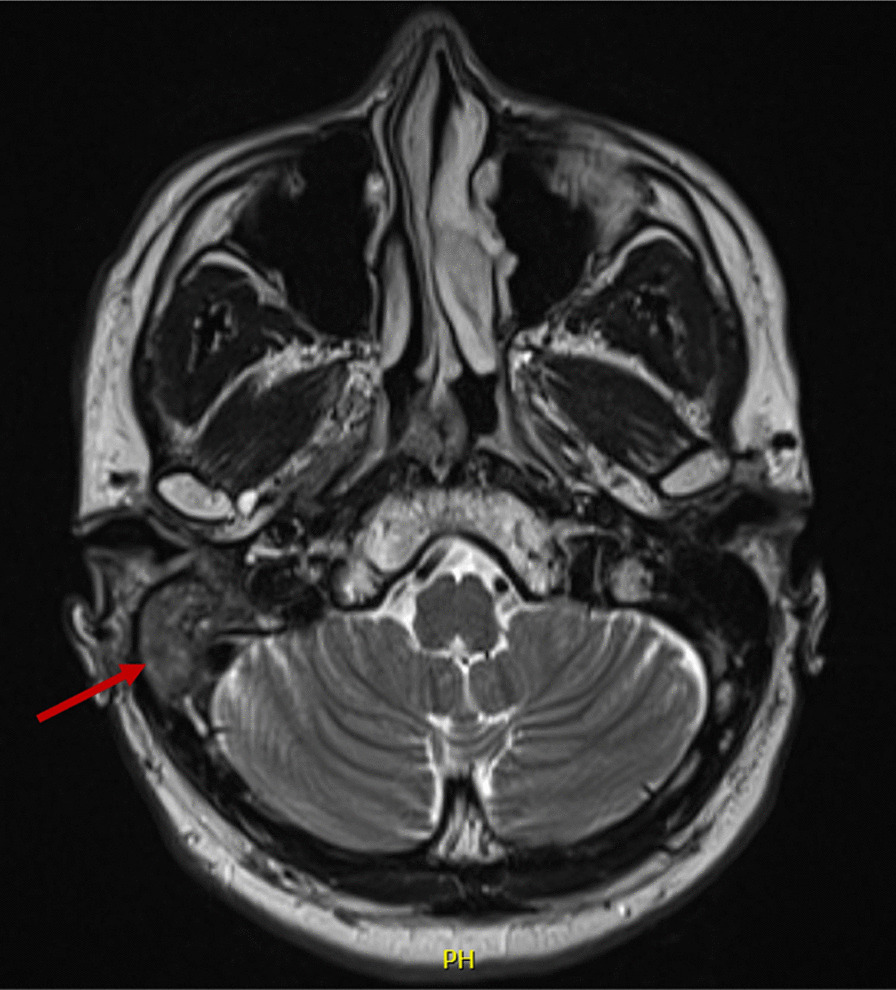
T2-weighted enhanced brain magnetic resonance imaging with arrow showing a right-sided soft tissue lesion within the mastoid cavity
